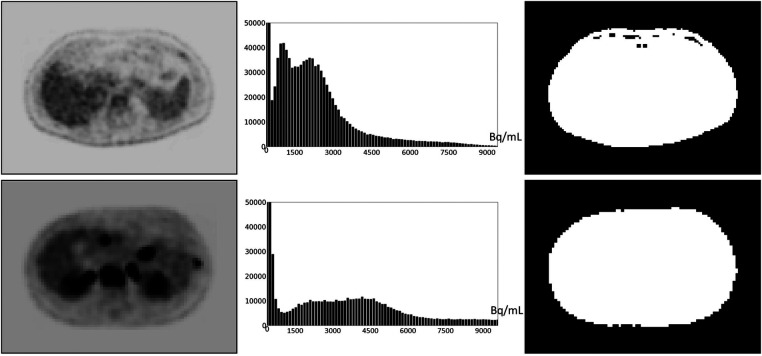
An FDG-PET axial image slice at the mid abdominal level selected from a 3D body torso image *I* (left), the histogram of the full 3D image *I* (middle), and the binary image (right) resulting from thresholding *I* at mean(*I*). (Top): Image from a normal subject. (Bottom): Image from a patient with metastatic cancer. For better visualization, some background regions are trimmed in the slice displays on the left.
